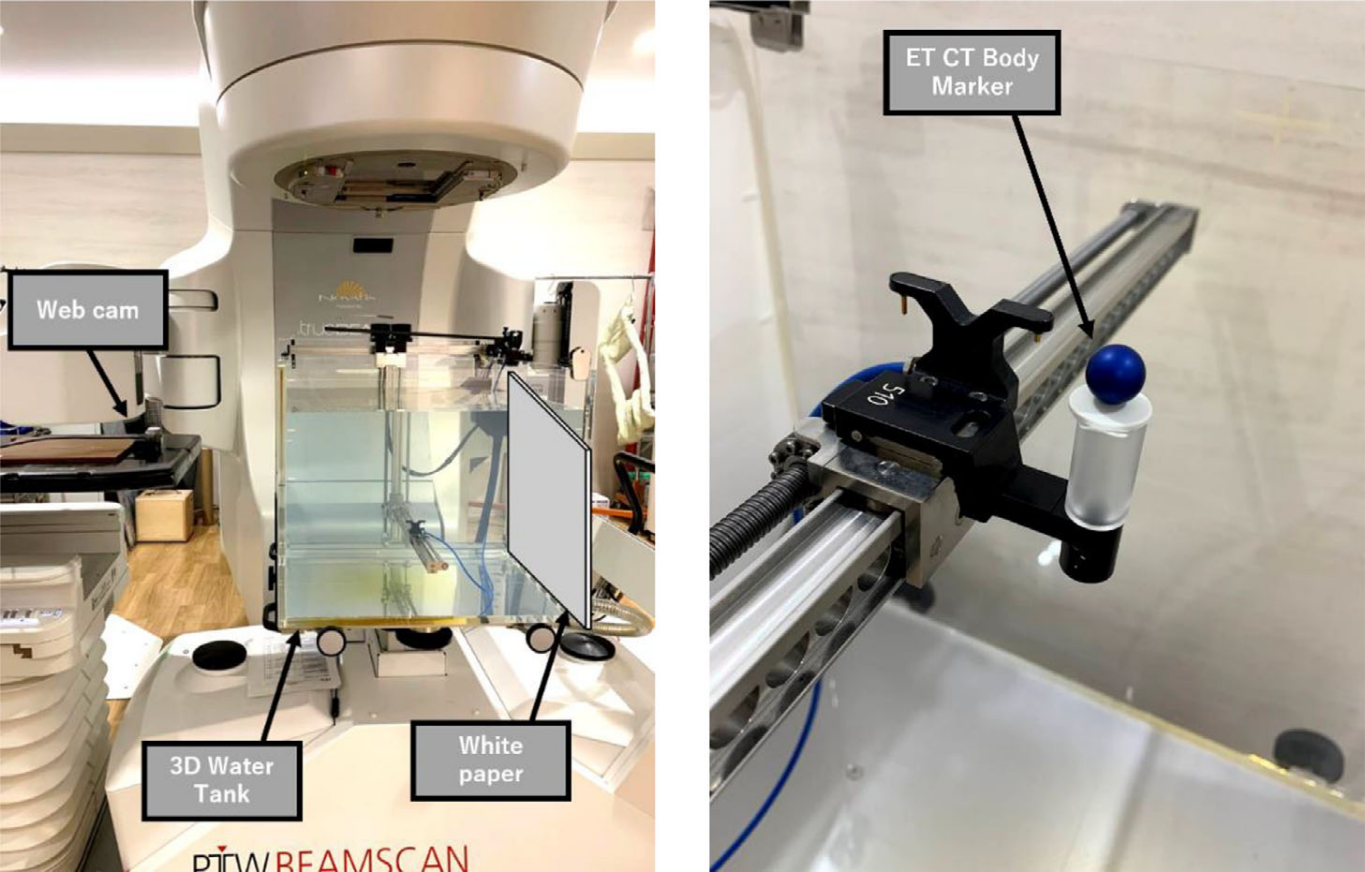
(a) Experimental setup for measuring the error between the indicated and measured drive speeds. (b) ET CT Body Marker attached to the drive unit.
